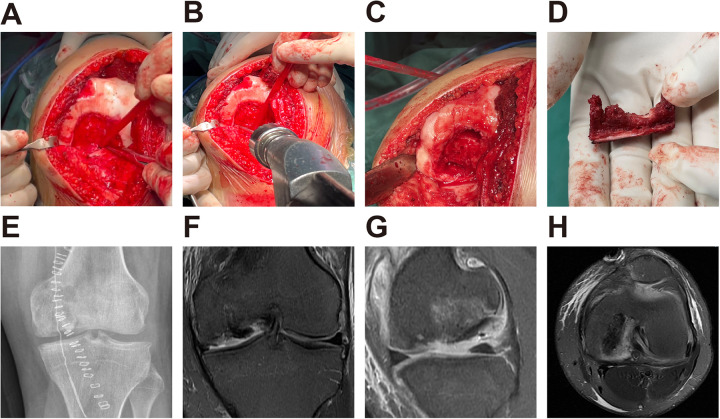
Intraoperative demonstration of autologous osteoperiosteal transplantation and x-ray and MRI after operation at 1 day. (A) Photograph of the osteochondral defect on the left medial femoral condyle. (B) Multiple drilling using a K-wire was performed toward the debrided lesion. (C) Bone sockets were made at the proximal and distal ends of the defect. (D) The ends of the graft are modified into protrusions. (E) Postoperative x-ray showed that the graft was in a good position. (F–H) MRI showed the defect was filled with the osteoperiosteal graft.
